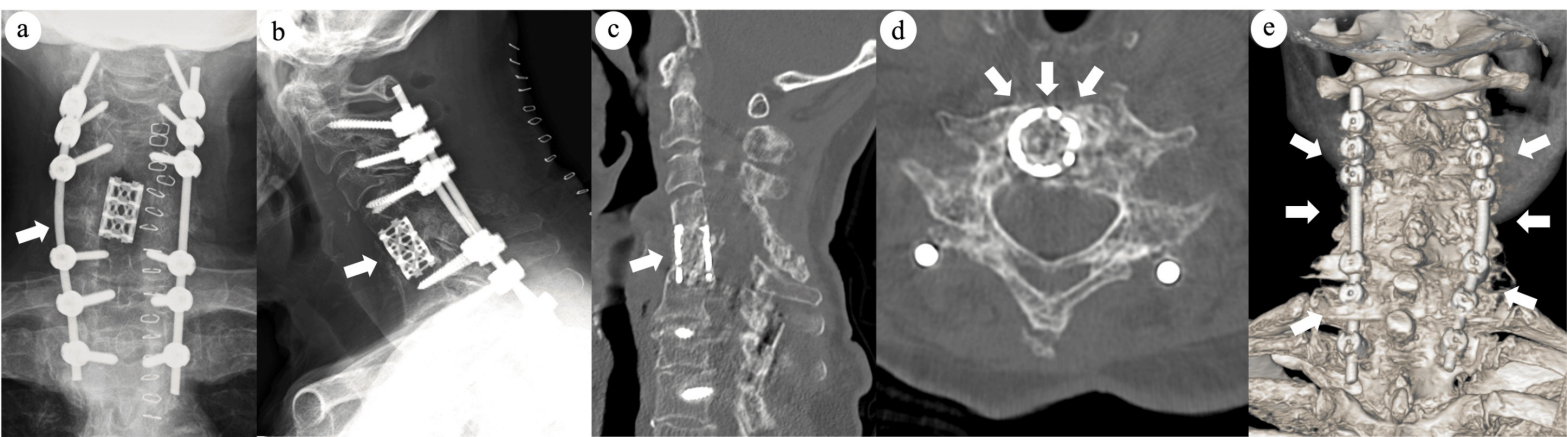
Radiological findings after surgery. Plain radiographs showing immediate postoperative (a) anteroposterior and (b) lateral views. (c) Sagittal, (d) axial, and (e) three-dimensional computed tomography scans obtained at four months postoperatively confirmed adequate decompression and bone fusion following posterior fixation with pedicle screws and reconstruction using a cylindrical titanium mesh cage with an autologous iliac bone strut (arrows).
